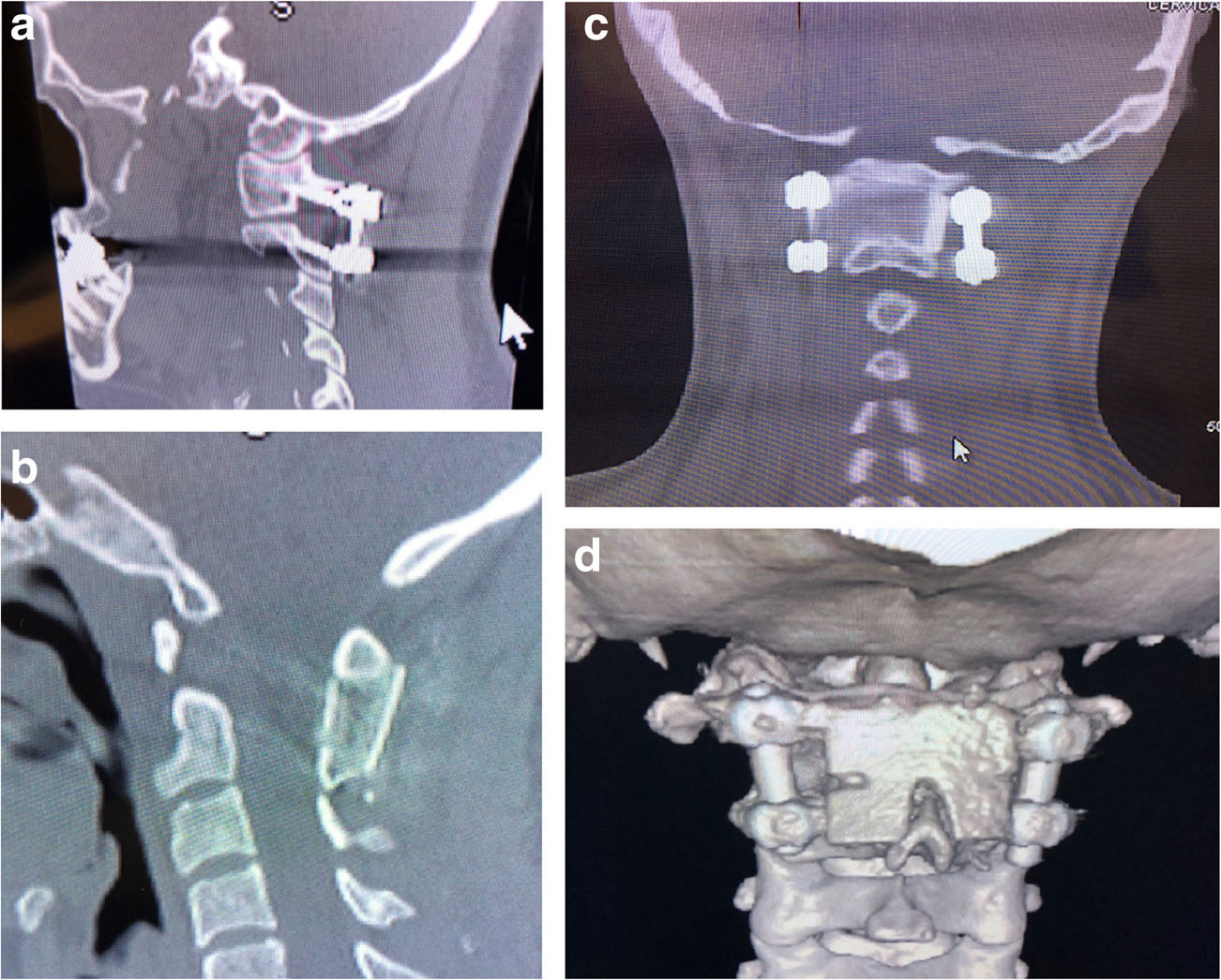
a Sagittal reconstruction of the cervical spine CT showing placement of the C1-C2 screws and connecting rods (Goel-Harms technique of C1-C2 stabilization). b CT mid-sagittal reconstruction showing the C1-C2 fusion. Note the allograft encompasses the posterior ring of C1 and tightly abuts the spinous process and lamina of C2. c Coronal view of CT showing graft placement between the posterior ring of C1 above and the spinous process and lamina of C2 below, and between the screws at C1-C2 bilaterally. d A 3D CT reconstruction of the upper cervical spine showing the C1-C2 fusion and stabilization from a posterior perspective.
